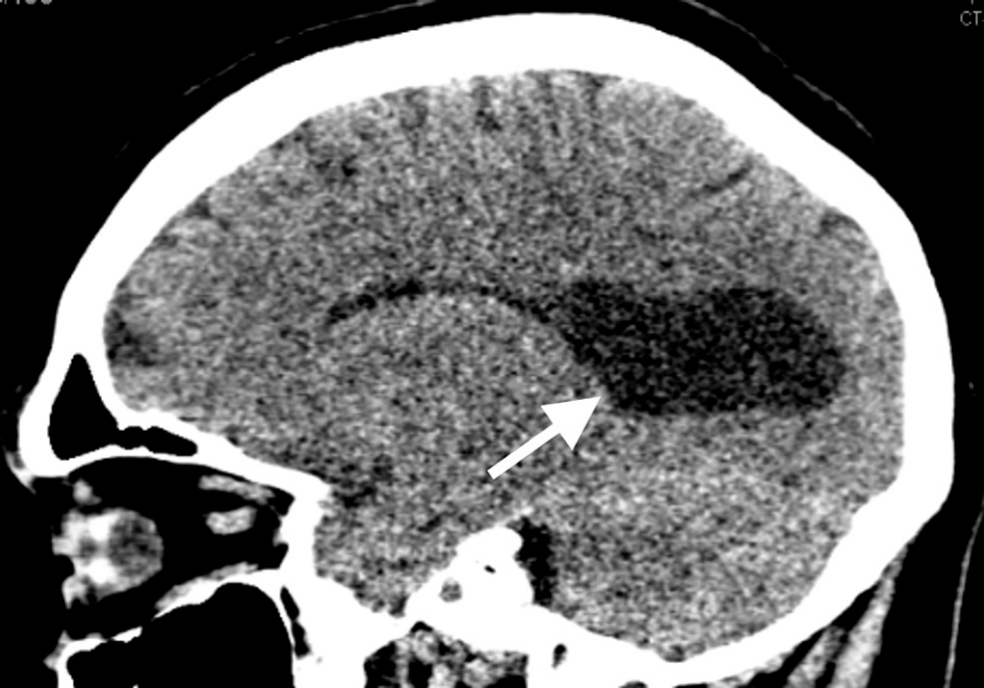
CT brain without contrast revealing colpocephaly (arrow) and absence of the corpus callosum.
